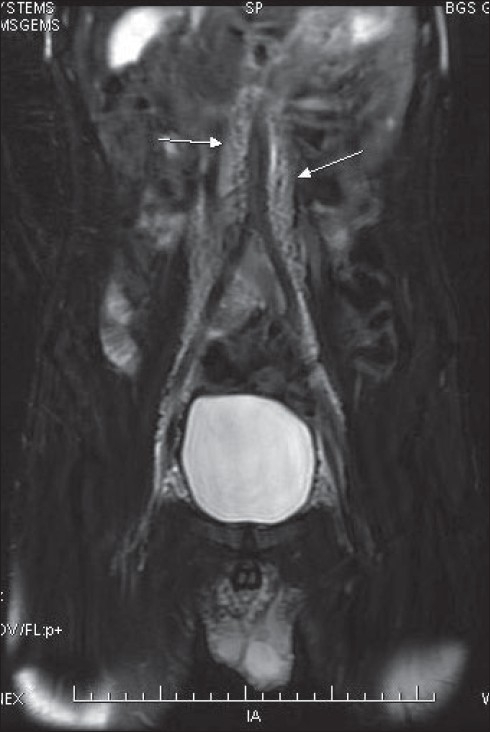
MRI scan of the abdomen and pelvis reveals paraaortic lymphangiectasia (arrow)
